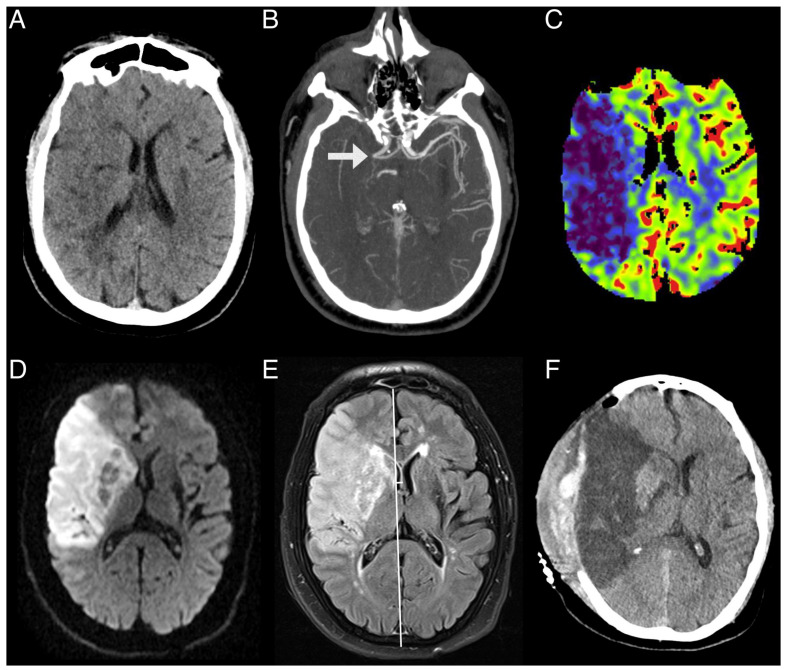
A previously healthy 55-year-old male transferred to the emergency department with severe right hemispheric syndrome (NIHSS: 20). NCCT (A) showed an extensive right ischemic lesion (ASPECTS 4) due to an occlusion of the M1 segment of the middle cerebral artery visualized on CTA ((B), arrow) and an extensive and severe volume drop on rCBV-based CTP, where the darker blue color indicates greater volume drops (C). Due to the extent of the injury, acute reperfusion treatment was not performed. The follow-up MRI performed 16 h after admission showed an extensive ischemic area on DWI (D), as well as abundant cerebral edema on FLAIR (E), causing a midline shift greater than 6 mm. A decompressive hemicraniectomy was performed 24 h after admission. The follow-up NCCT revealed an extensive ischemic lesion with cerebral edema and postsurgical changes (F). After the procedure, the patient survived but with serious sequelae that led to severe disability at 3 months.
